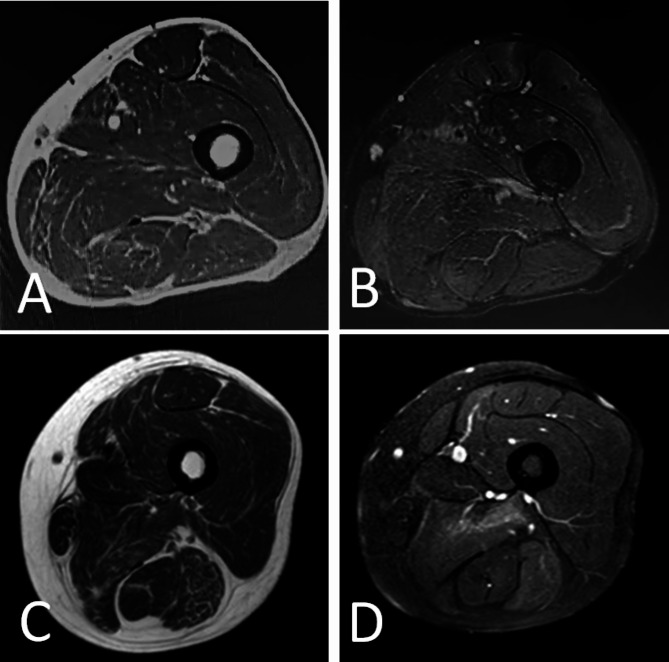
Right thigh MRI of 2 cases. Axial T1WI (A) and STIR (B) images of case 1, and axial T1WI (C) and STIR (D) images of case 2were shown
